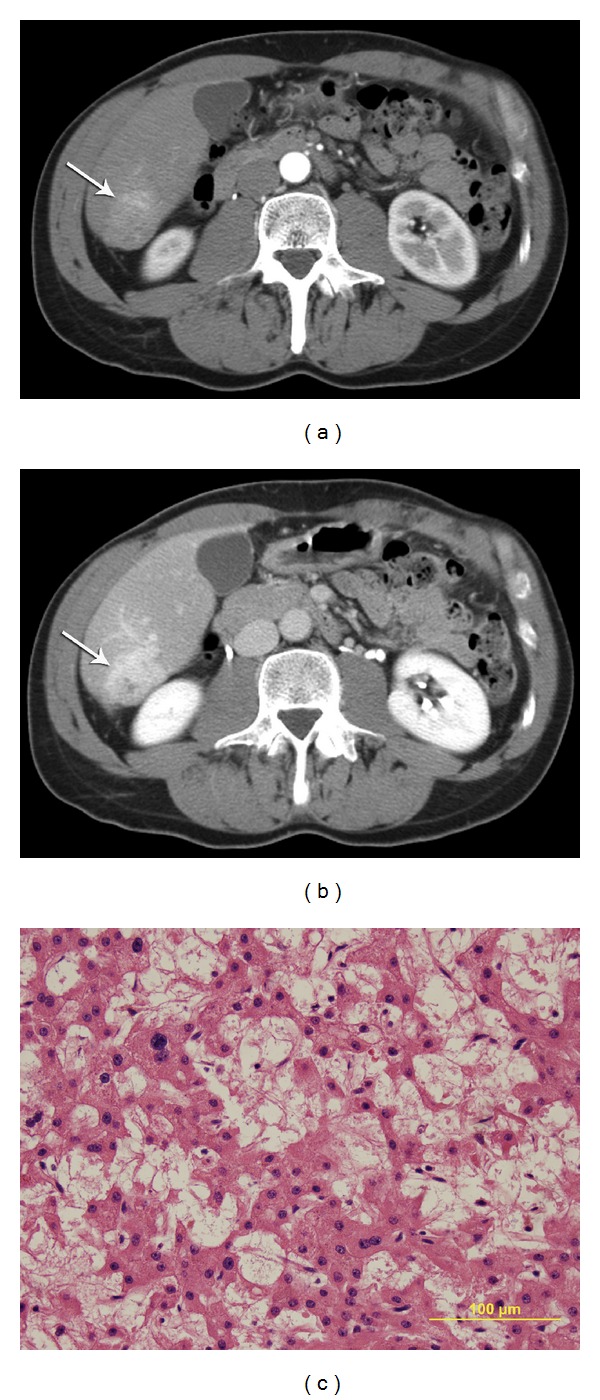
Case  7. History: 51 year-old-male with no cirrhosis, AFP 1.8, and no hepatitis. At surgery this was pathologically proven to represent liver adenoma. (a) Arterial phase CT demonstrating enhancing mass with lobulated margin (arrow). (b) Delay images demonstrating persisting enhancement (arrow) with focal hypodense area noted centrally. This was considered an equivocal mass on review. (c) Fine needle aspiration biopsy with three passes and three core biopsies demonstrated epithelial neoplasm and adenoma versus HCC. This was reviewed at an outside institution with the diagnosis of well-differentiated HCC. The smears are cellular and show hepatocytes with minimal atypia in sheets and small clusters. Prominent capillaries are noted within the sheets and endothelial cells are noted to be encircling clusters of hepatocytes. Post-surgery pathology revealed this to be adenoma.
